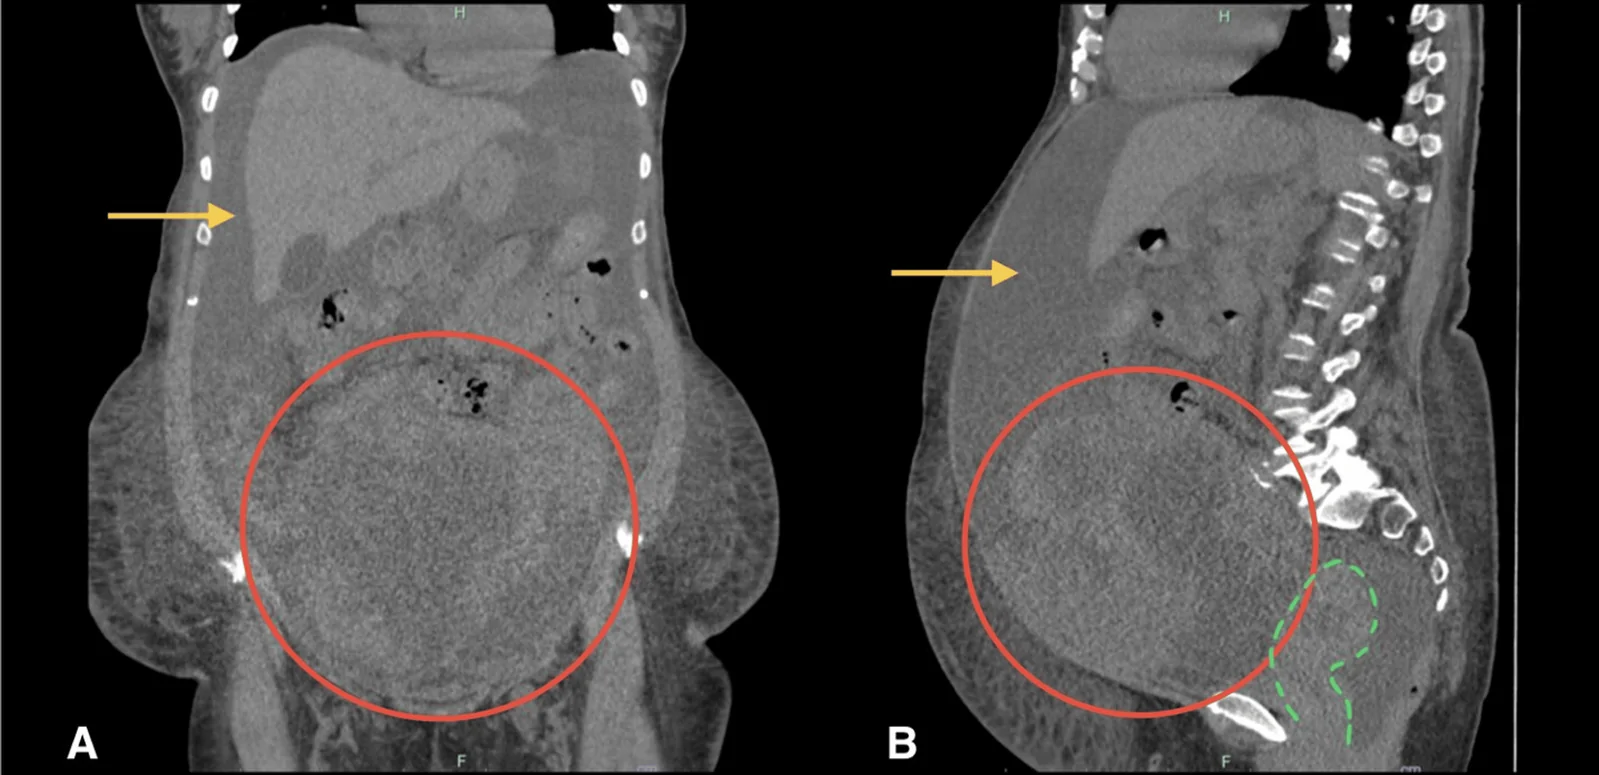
Contrast-enhanced computed tomography (CT) of the abdomen and pelvis Demonstrates a large heterogeneous adnexal mass (red circle). (A) Coronal CT image shows the lesion occupying the pelvis with associated ascitic fluid (yellow arrow); (B) Sagittal CT image confirms the extent of the mass, with ascitic fluid and the uterus (outlined by the green dashed line).
